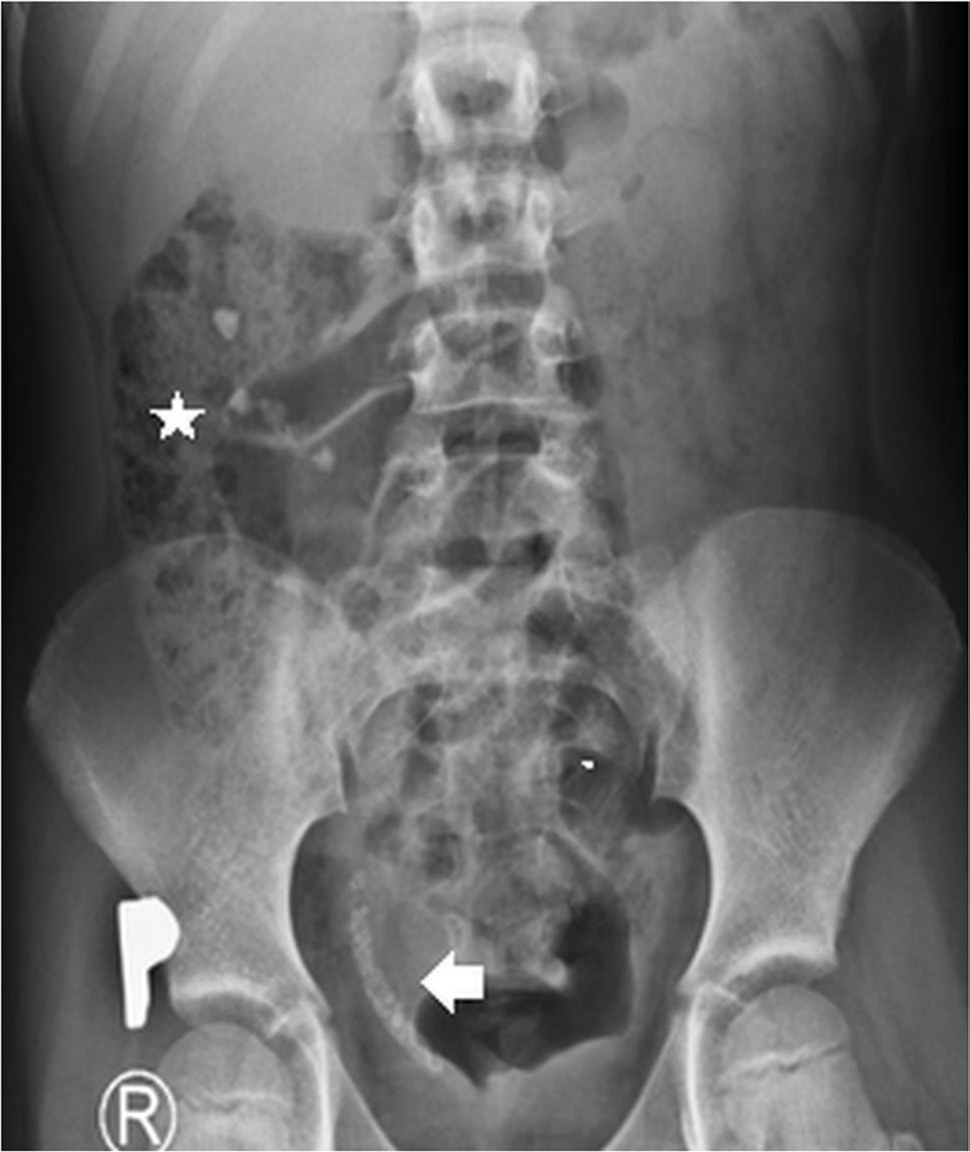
“Steinstrasse” in a 12-year-old boy. Anteroposterior kidney, ureter and bladder radiograph shows steinstrasse, or a column of stone fragments in the distal part of the right ureter (*arrow*) as a complication of extracorporeal shock wave lithotripsy. Note stone fragments in the kidney and right ureter (*asterisk*). Case courtesy of Prof. Philippe Petit, Marseille, France
